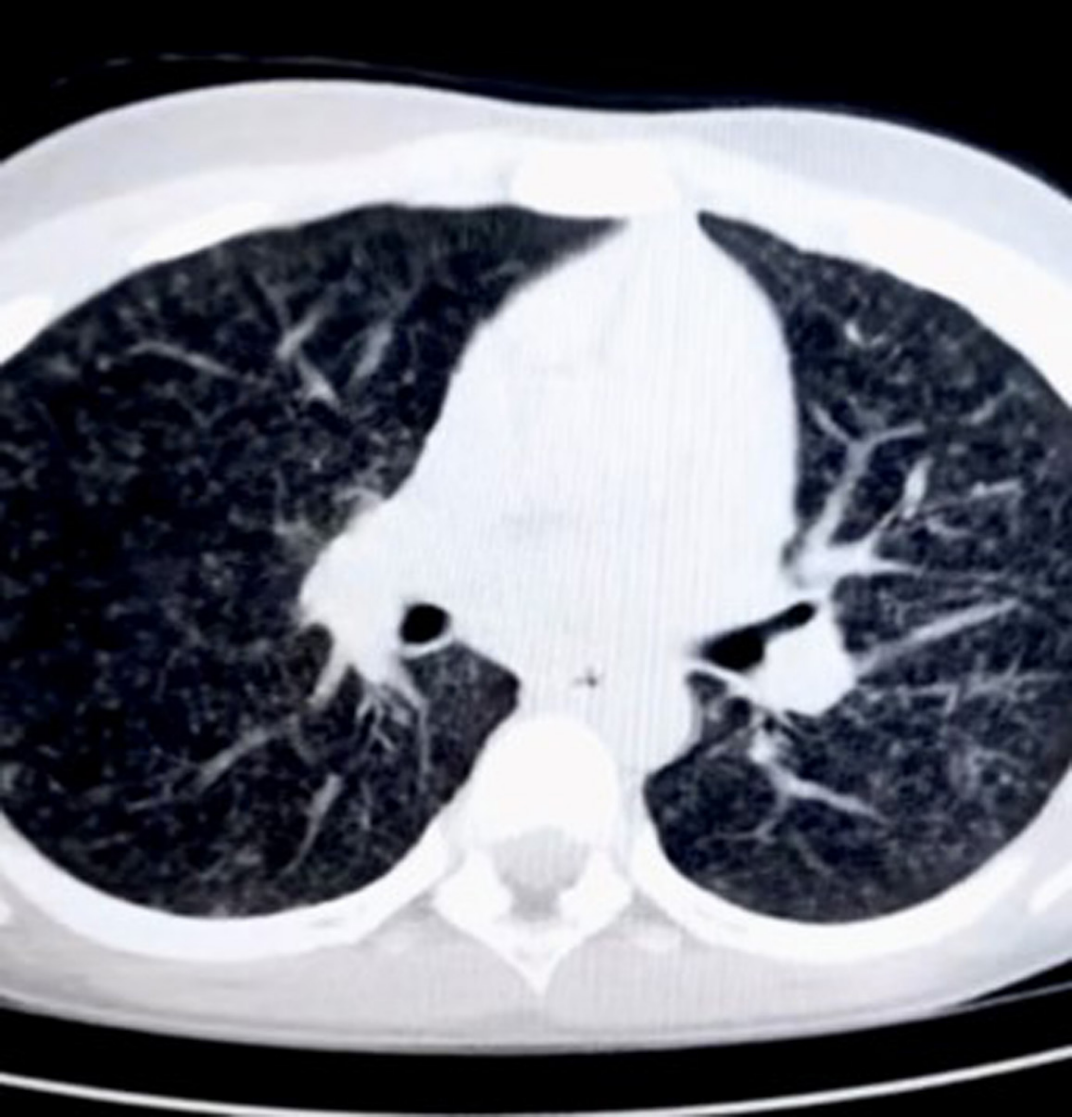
HRCT showing numerous tiny (2–3 mm) nodules in both lungs, with branching patterns in both the upper and lower lobes.
